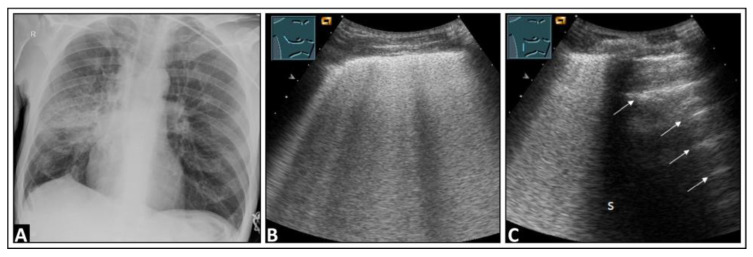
A patient with dyspnea, cough, elevated inflammatory markers, and right-sided pneumonia in the mid-zone observed on a chest X-ray (A). (B) On lung ultrasound, multiple confluent B-lines are visible, indicative of a “white lung” pattern. (C) There are a few intercostal spaces deeper than in panel B, there is an increase in B-lines cranially, and a normal lung is observed caudally with corresponding A-lines (arrows), similar to a localized interstitial pattern; dorsal sound extinction (s) is observed behind a rib.
